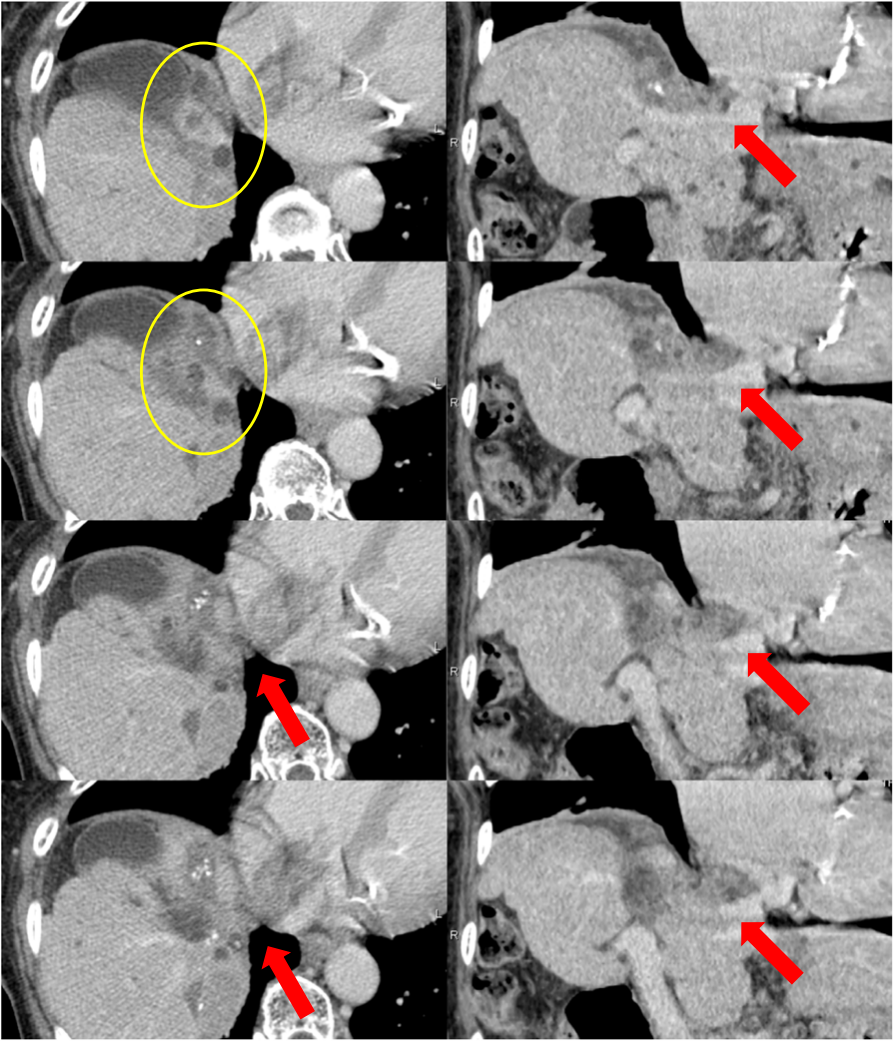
Dynamic-enhanced computed tomography imaging of case 3. Tumor thrombus was detected in the right hepatic vein, inferior vena cava (IVC), and right atrium (RA; red arrow), as well as in the right phrenic vein (yellow circle). The left column shows the axial view, and the right column shows the coronal view
